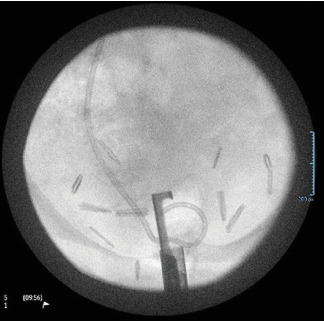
X-ray of Mauermayer stone crusher catheter extraction.
